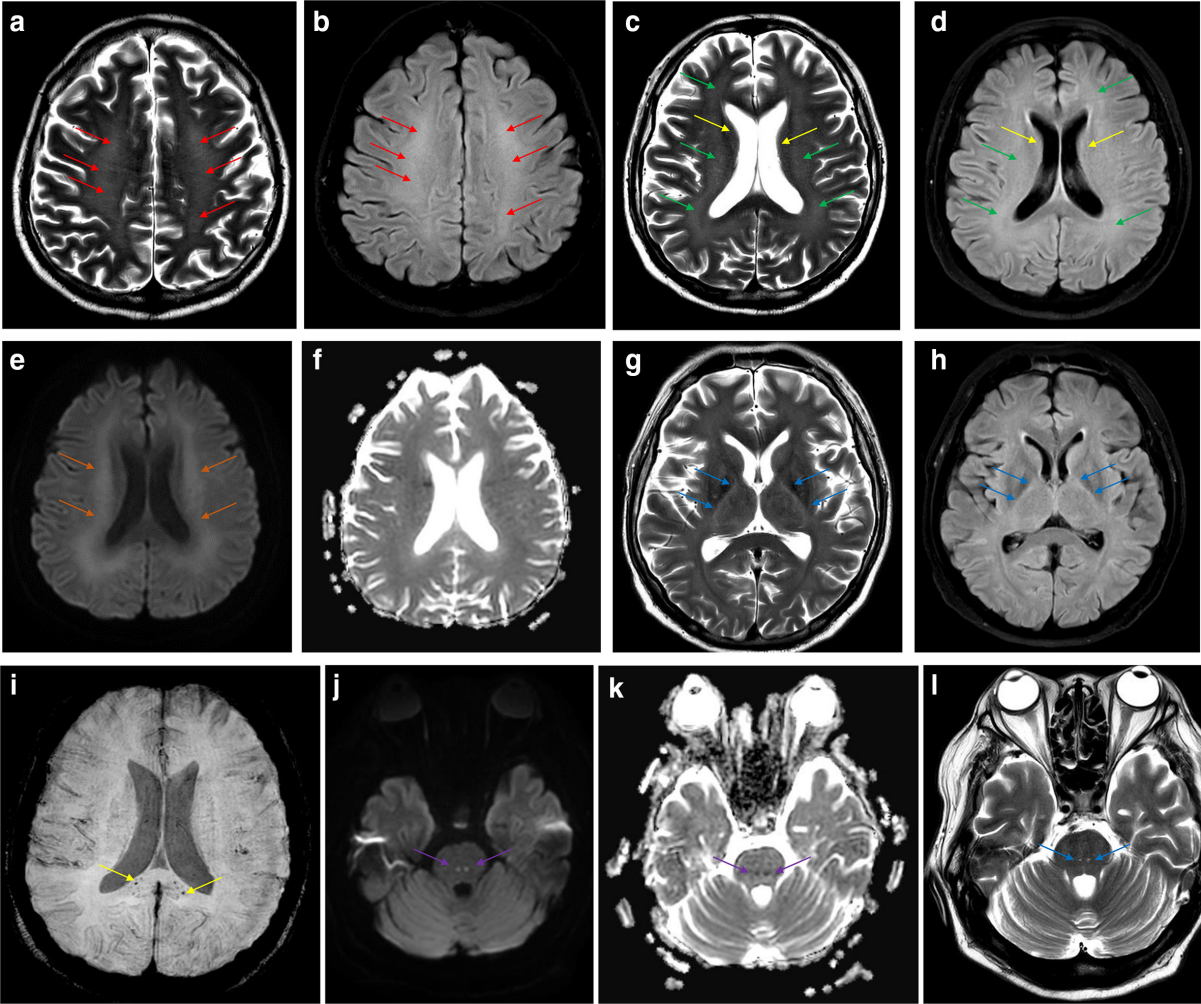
Axial T2W image (a) and FLAIR image (b) at the level of centrum semiovale showing bilateral symmetric centrum semiovale faint high signal intensity (red arrows). Axial T2W image (c) and FLAIR image (d) at the level of body of lateral ventricles show bilateral symmetric periventricular (yellow arrows) and corona radiata (green arrows) faint high signal intensity. Axial diffusion-weighted (e) and ADC images (f) at the level of lateral ventricle bodies showing corresponding regions of diffusion restriction (high DWI signal and low ADC value) at corona radiata bilaterally (orange arrows). Axial T2W image (g) and FLAIR image (h) at the level of basal ganglia show bilateral symmetric high signal intensity at posterior limb of both internal capsules (blue arrows). Susceptibility-weighted images (SWI) image (i) at the level of body of lateral ventricles showing minute dark blooming foci at splenium of corpus callosum (yellow arrows). Axial diffusion-weighted (j), ADC images (k) at the level of pons showing two bilateral symmetric foci of diffusion restriction (high DWI signal and low ADC value) involving reticular formation region (purple arrows). Axial T2W image (l) at the same level show two bilateral symmetric foci of high signal intensity in the same corresponding locations in reticular formation (blue arrows).
